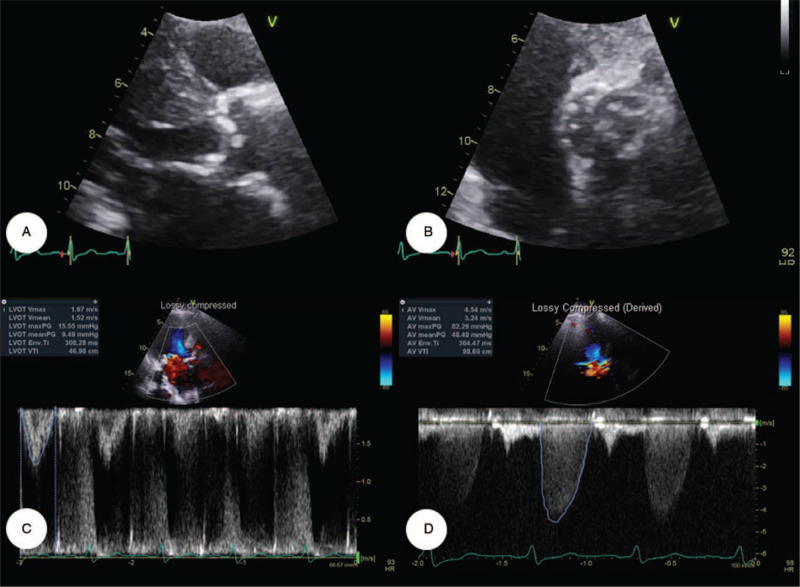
Initial transthoracic echocardiography. (A, B) Parasternal long- and short-axis views revealed a heavily calcified aortic valve. (C, D) The peak velocity was 4.54 m/s and the mean pressure gradient was 48.5 mm Hg, suggestive of severe aortic stenosis.
